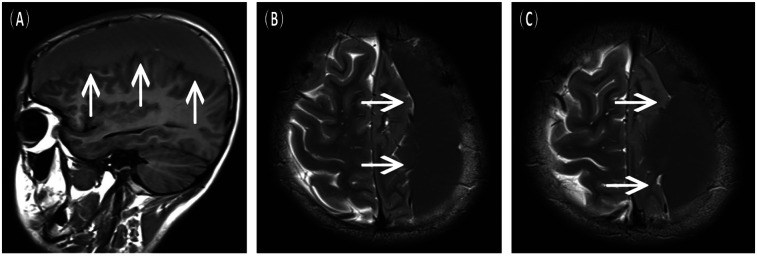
Preoperative MRI scans upon the current admission, indicative of a left chronic subdural hematoma. (A) Sagittal view, T1-FLAIR sequence. (B,C) Axial view, T2-weighted sequence.
